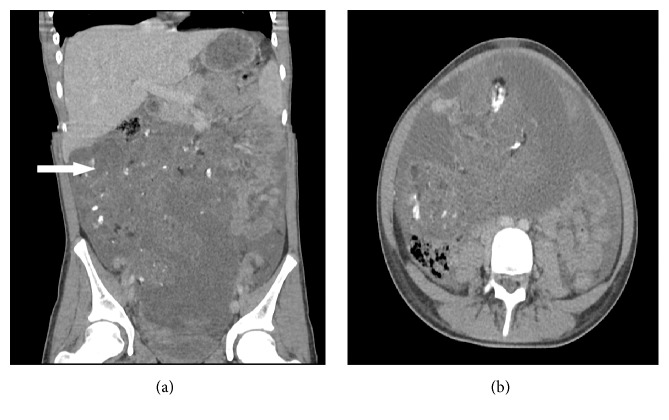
Initial imaging computed tomography of the abdomen and pelvis demonstrating a large pelvic tumor (arrow).
